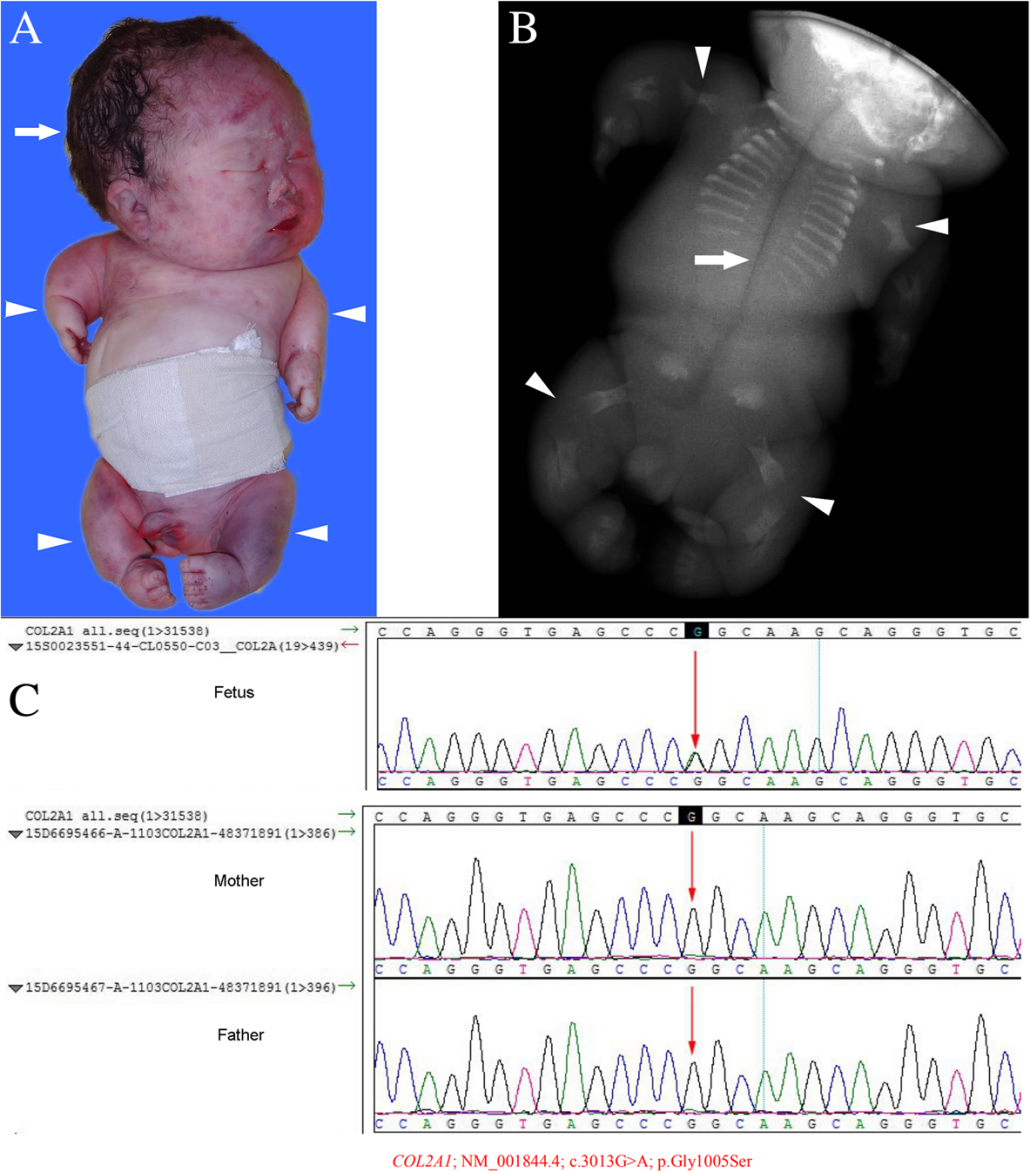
Case 19: The fetus with achondrogenesis. a Gross anatomy of the aborted fetus, showing the markedly short arms and legs (arrowheads), with a giant skull (arrow). b X-ray images of the aborted fetus, showing the severe short limb (arrowheads), and the defective ossification of the spine, sciatic, pubic and iliac bone (arrow). c The fetus carried the c.3013G > A (p.Gly1005Ser) mutation in the *COL2A1* gene. The Sanger verification revealed that this mutation was new, and was not carried by the parents
